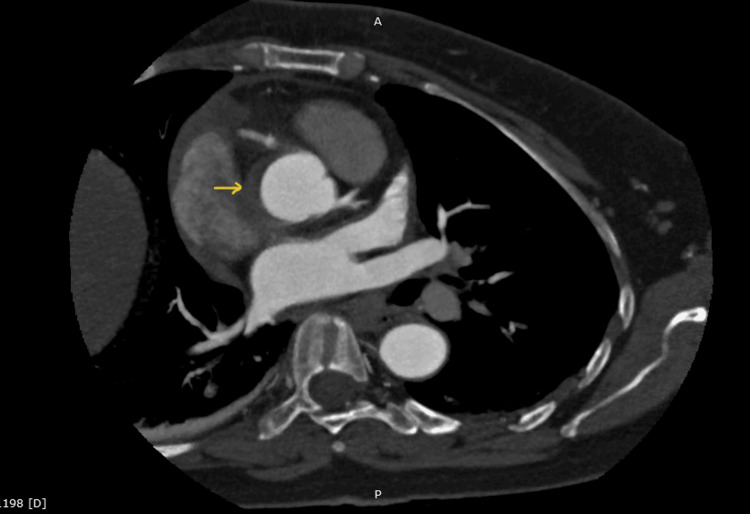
CT aortogram at the one-week follow-up showing the nearly resolved and stable dissection flap (arrow) with wide open true lumen of the ascending aorta
